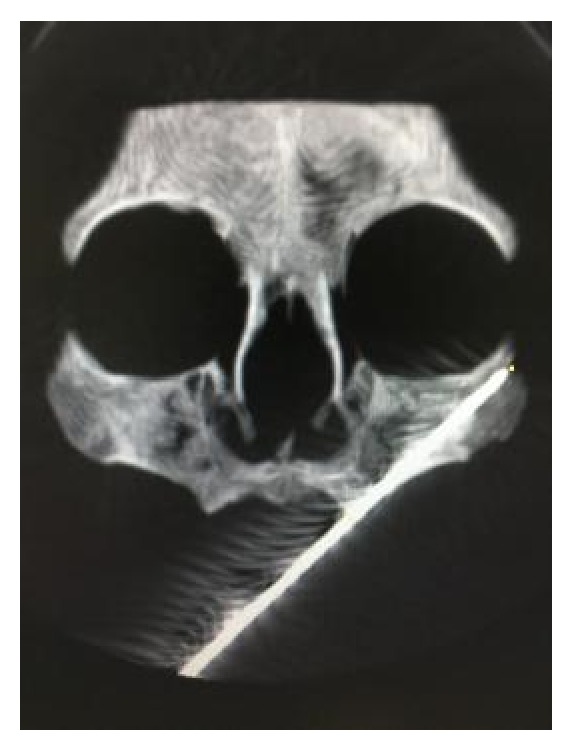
The implantation path on dry skull under CT scanning.
